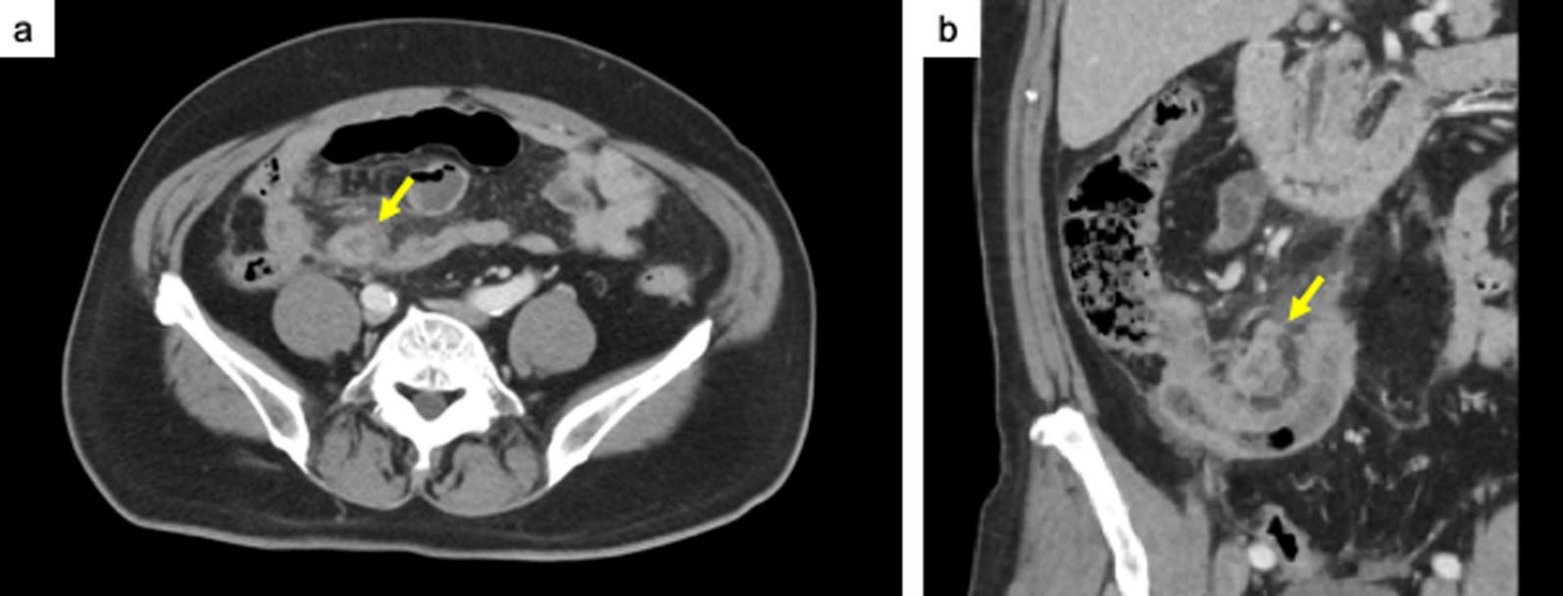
Abdominal computed tomography reveals acute appendicitis with swelling at the tip of the appendix and surrounding edema (yellow arrow)
